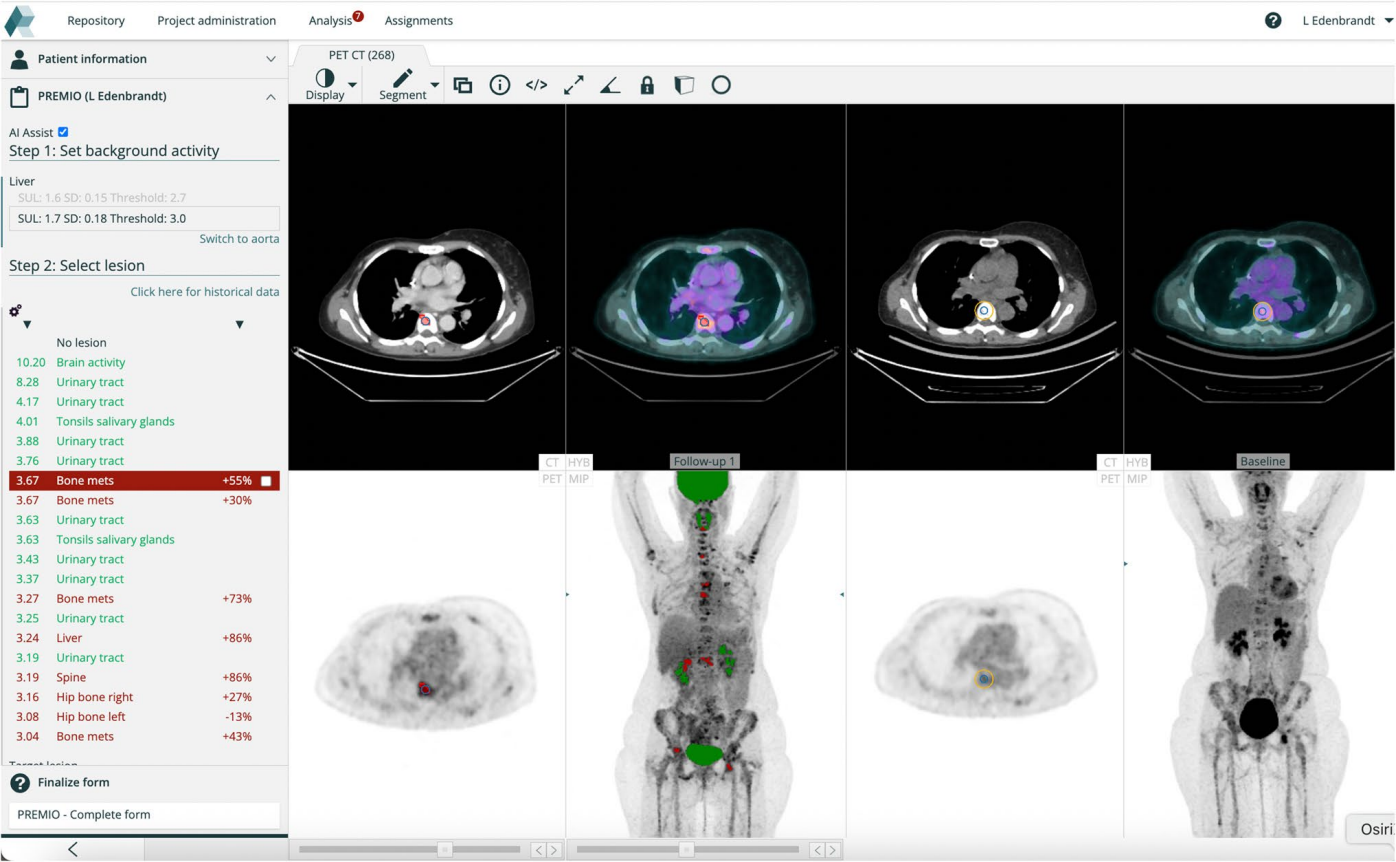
The coPERCIST module on the RECOMIA platform with baseline (right) and follow-up PET-CT (mid). All hotspots are compared between the scans and sorted according to SULpeak or percentage change in the left panel. Normal uptake is shown in green and lesions in red
